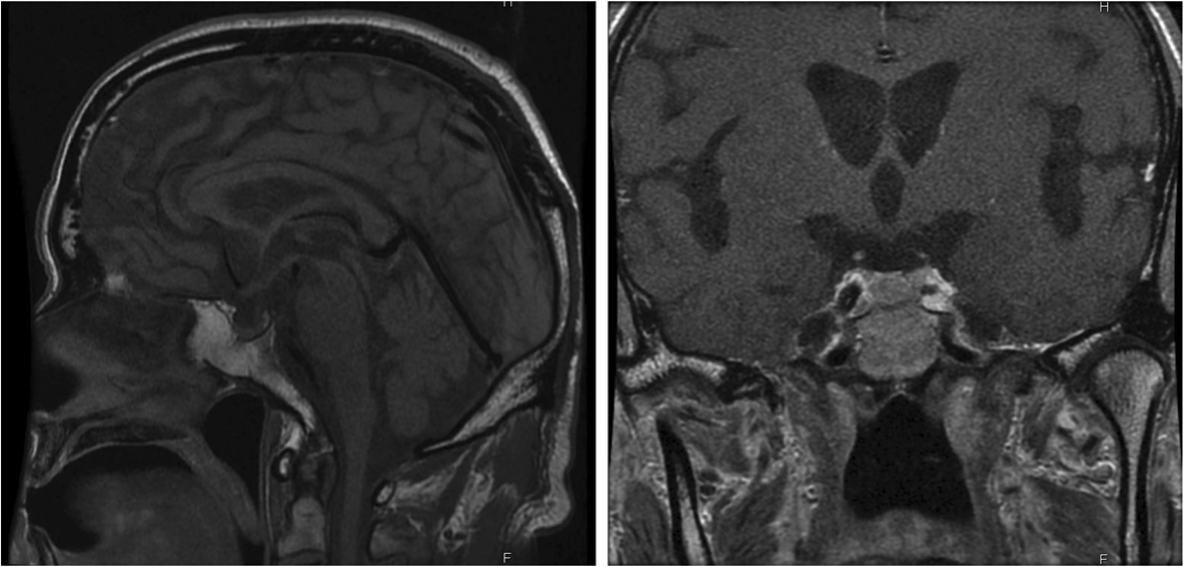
Magnetic resonance imaging after the onset of isolated adrenocorticotropic hormone deficiency. Magnetic resonance imaging showed no space-occupying lesions in the pituitary gland or hypothalamus.
